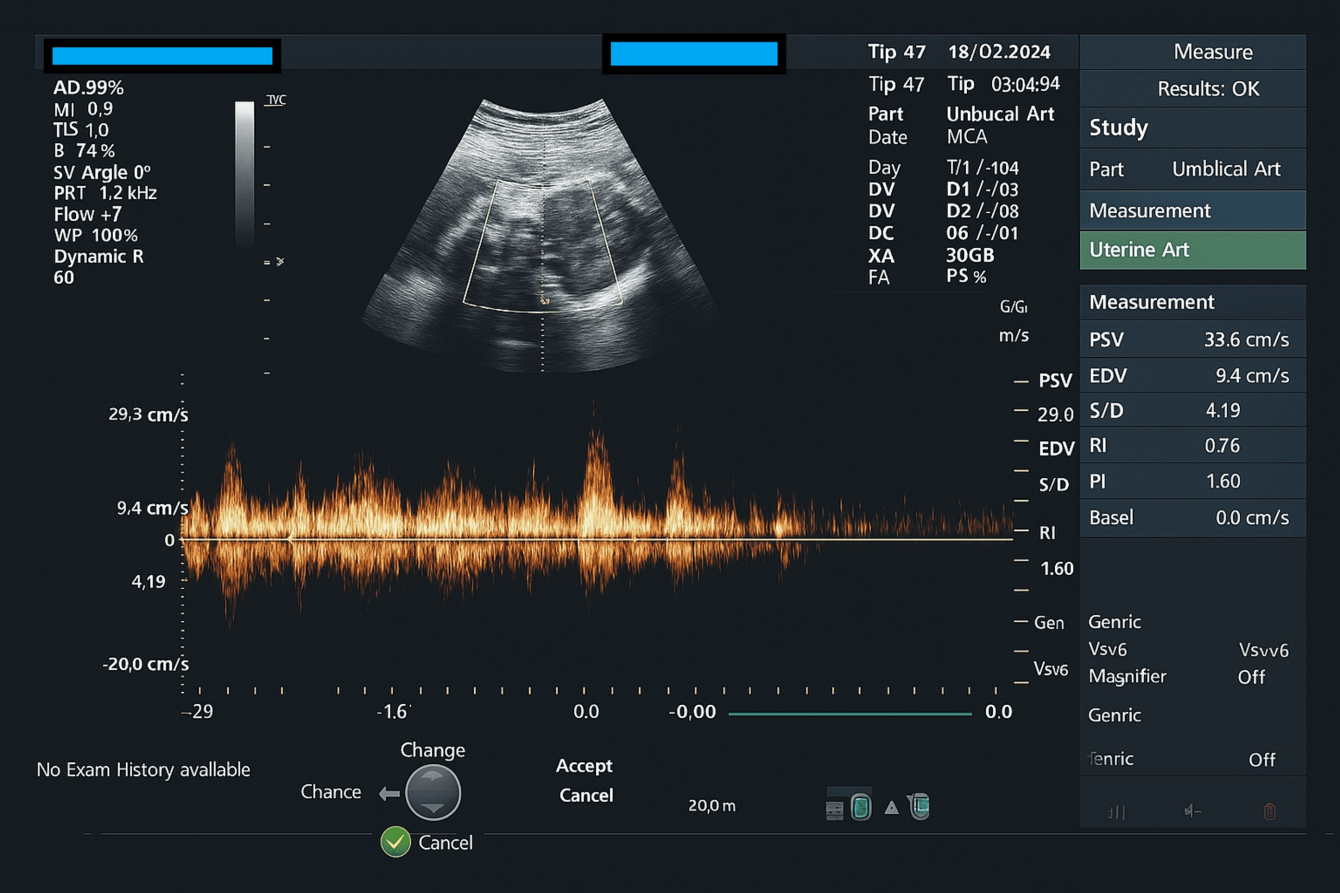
Abnormal Doppler of the middle cerebral artery of the fetus infected with CMV CMV: Cytomegalovirus
